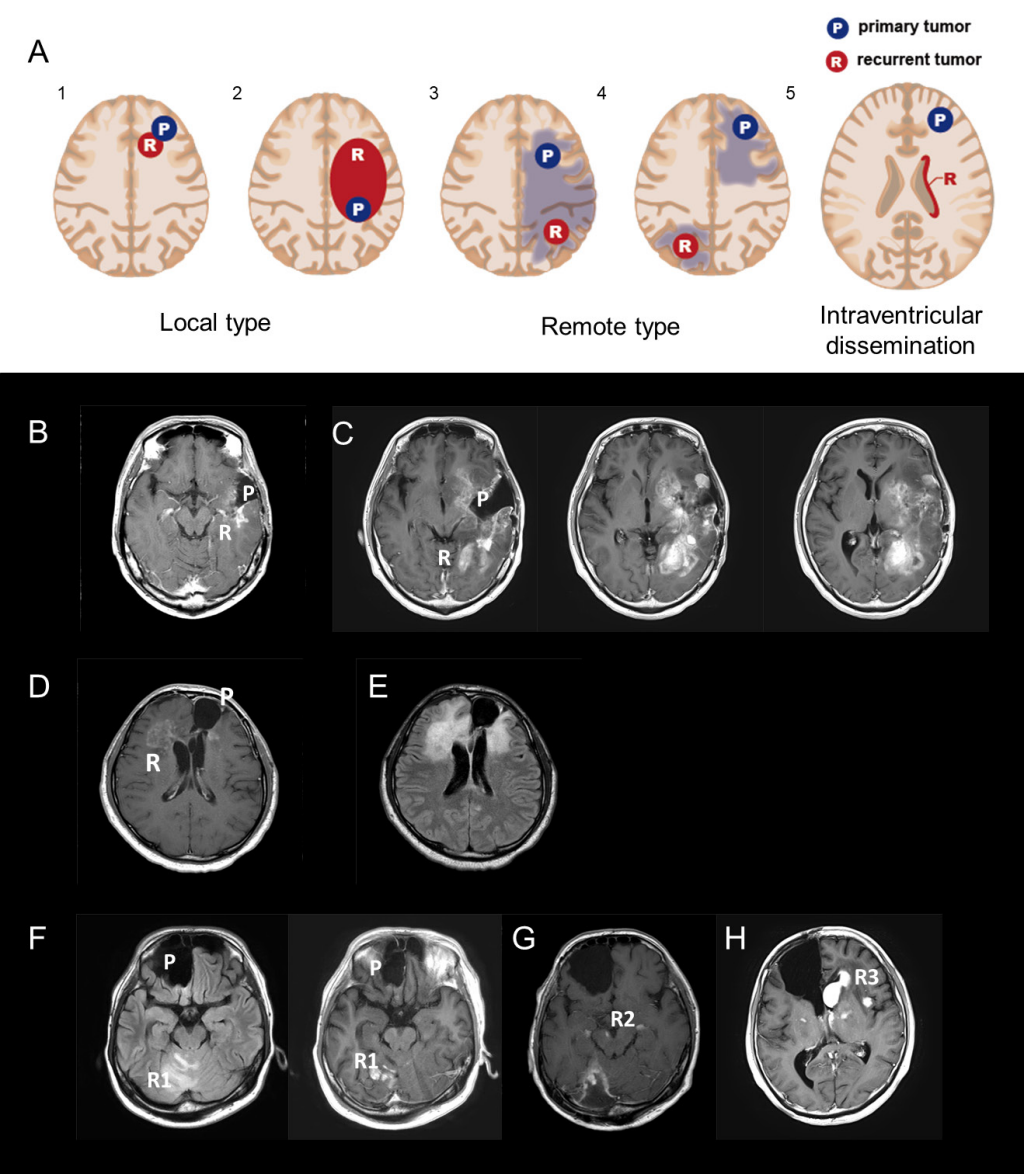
A. Five radiological recurrent patterns of gliomas: Type 1: local recurrence at the site of the primary lesion inside a single lobe; Type 2: diffuse enlargement from a primary lesion into different lobes; Type 3: an independent mass located in a different lobe, cerebellum, or brainstem that is continuous with the primary lesion in a T2/FLAIR high-intensity area; Type 4: a completely independent mass in a different lobe, cerebellum, or brain stem apart from the original lesion; and Type 5: intraventricular dissemination. B and C. A representative case of recurrent 1p/19q co-deleted glioma of Types 1 and 2 (W5). An MRI image (Gd-T1) taken 90 months after the first surgery (B); a recurrent contrast-enhanced tumor located around the cavity of the resected original tumor. At the time, the recurrent tumor was categorized as Type 1. MRI images (Gd-T1) after 115 months show a recurrent enlarged tumor located within the frontal and occipital lobes (C). D and E. A representative case of a *TP53* mutant glioma of Type 3 (M14). A Gd-enhanced independent tumor was detected from a primary lesion (D). A FLAIR image reveals two lesions that are continuous with a high-intensity area (E). F-H. A representative case of a *TP53* mutant glioma of Type 4 (M8). MRI images (FLAIR and Gd-T1) taken 61 months after the first surgery (F); an independent recurrent tumor, located in the right cerebellum, was detected apart from the primary tumor located in the right frontal lobe. An MRI image (Gd-T1) after 95 months shows that the recurrent tumor metastasized to the midbrain and cerebral peduncles (G). An MRI image (Gd-T1) after 100 months reveals that the recurrent tumors metastasized bilaterally to the basal nuclei (H). Abbreviation: Gd gadolinium.
